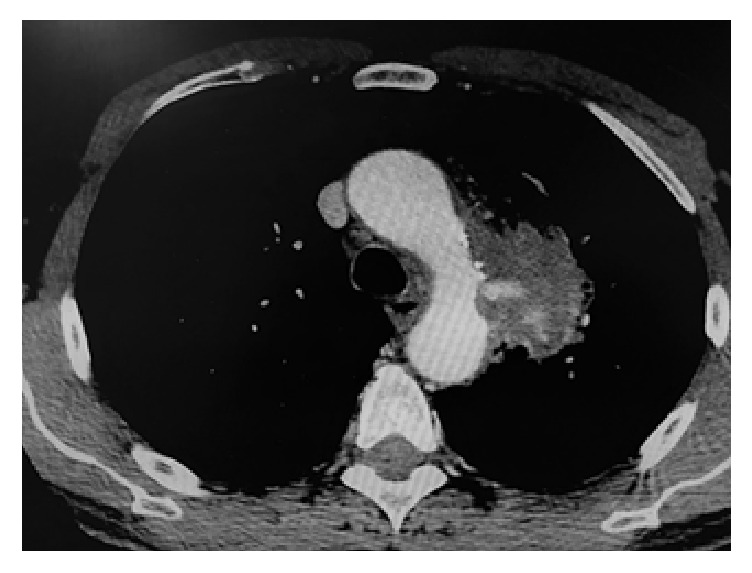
CT depicted an aortobronchial fistula with intrapulmonary hematoma.
